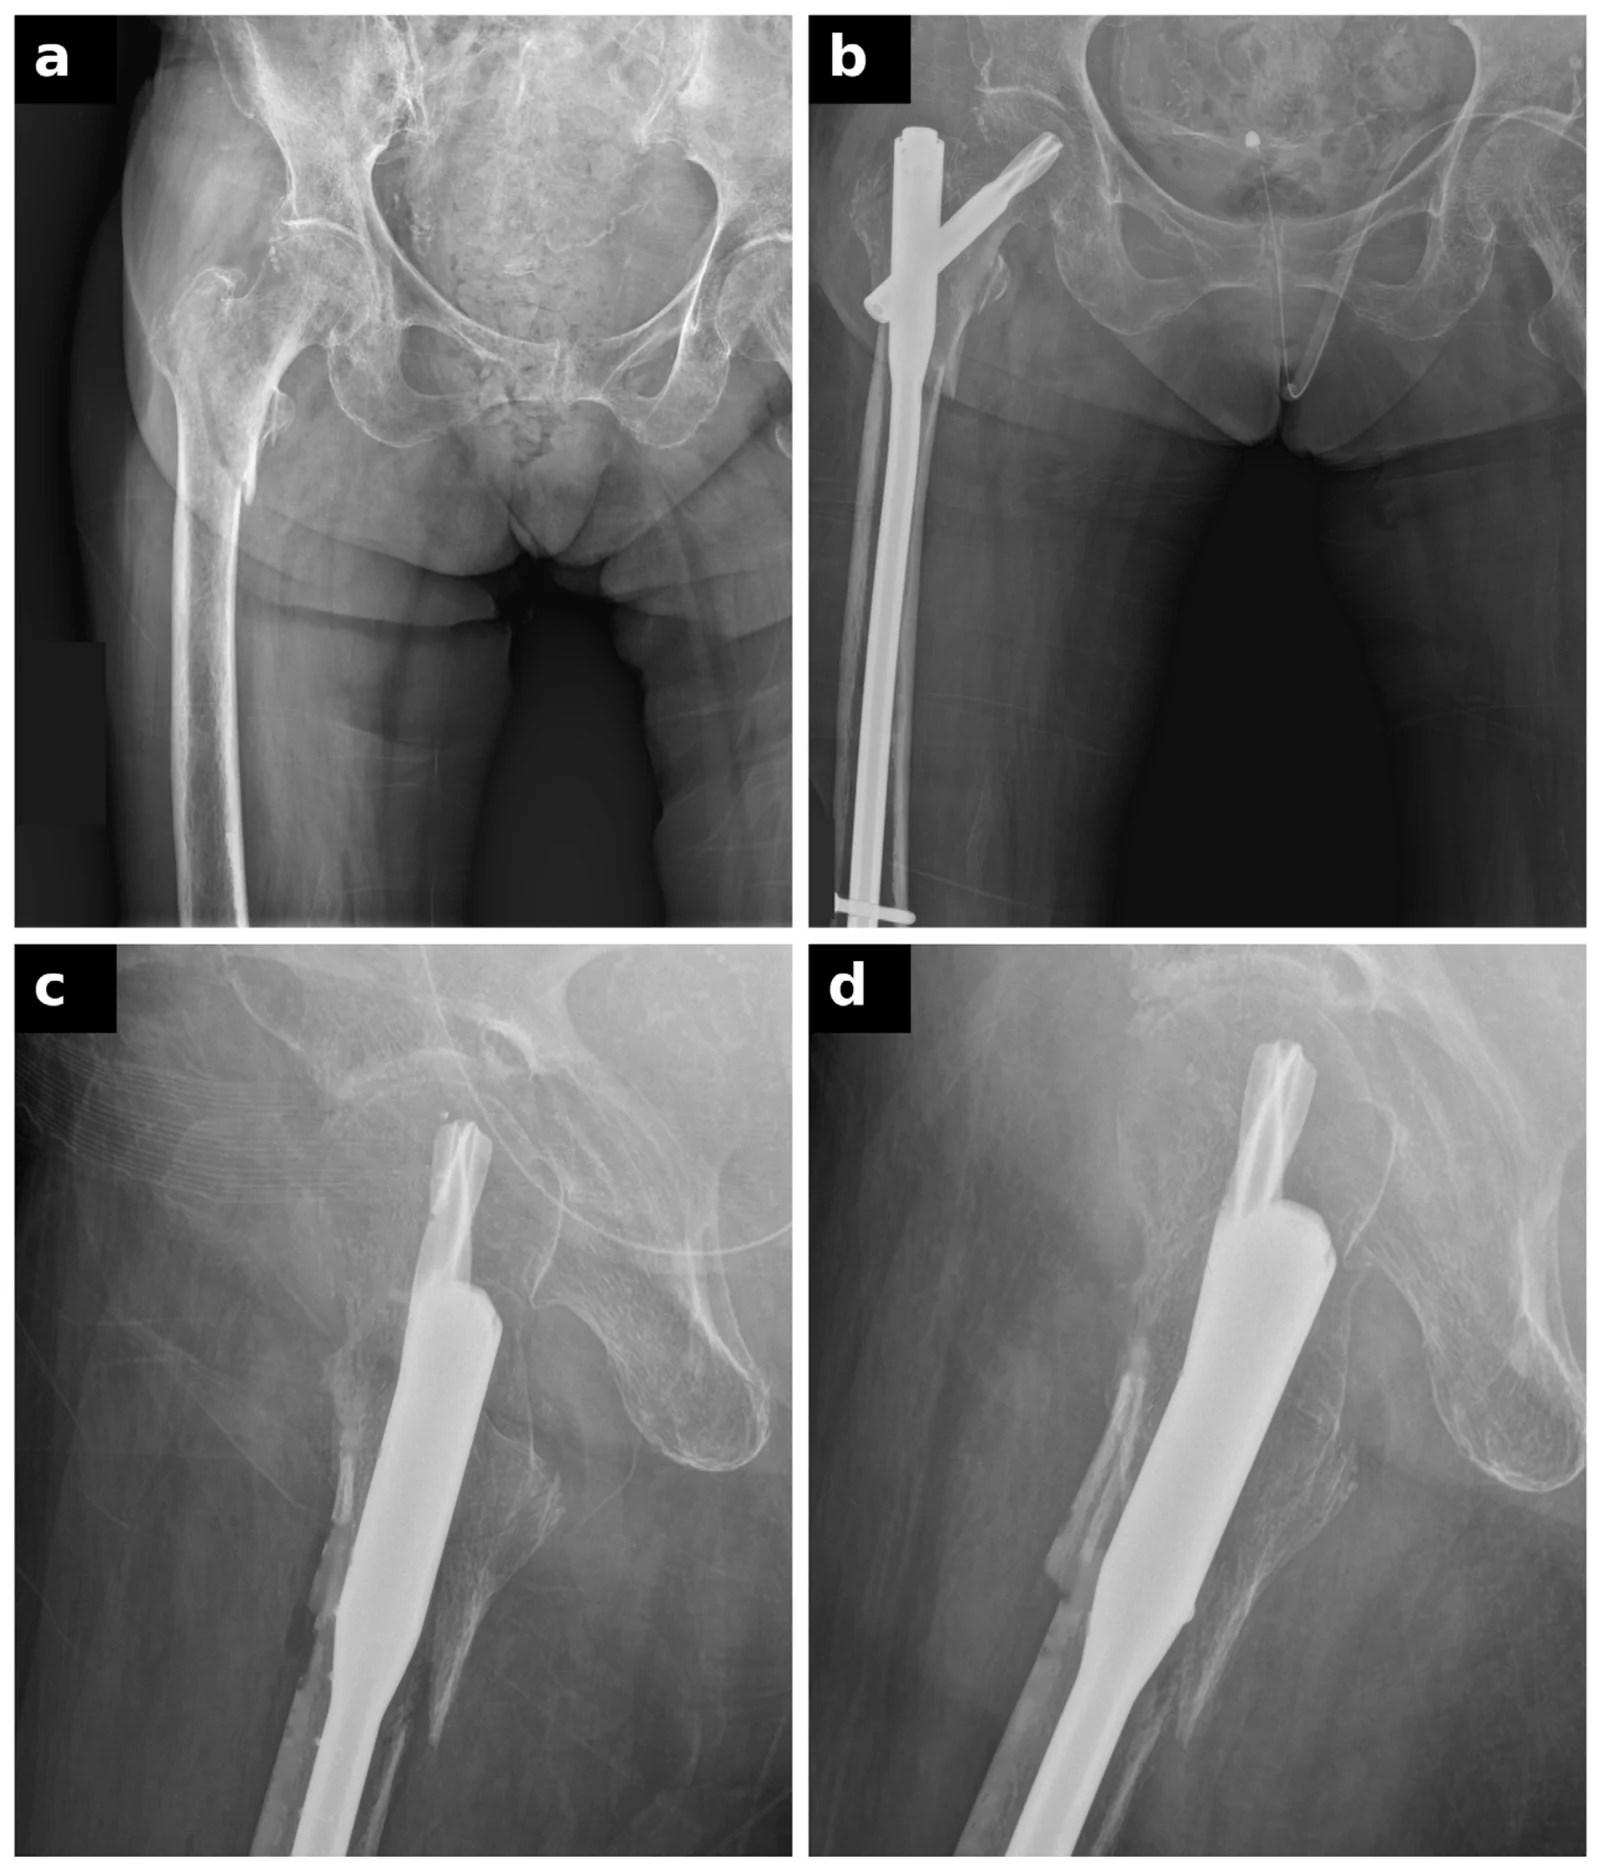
An 84-year-old woman with an AO/OTA 31-A2 fracture with a big lesser trochanteric fragment treated with a long cephalomedullary nail. (a) Preoperative anteroposterior radiograph. (b) Immediate postoperative anteroposterior radiograph (day 0), showing the whole nail passing the femoral isthmus with two distal interlocking screws. (c) Immediate postoperative lateral radiograph (day 0), used as the baseline. (d) Lateral radiograph at the first outpatient visit (4 weeks after surgery): anterior cortical contact is maintained without posterior sagging. Panels (a–d) are shown at the same magnification.
